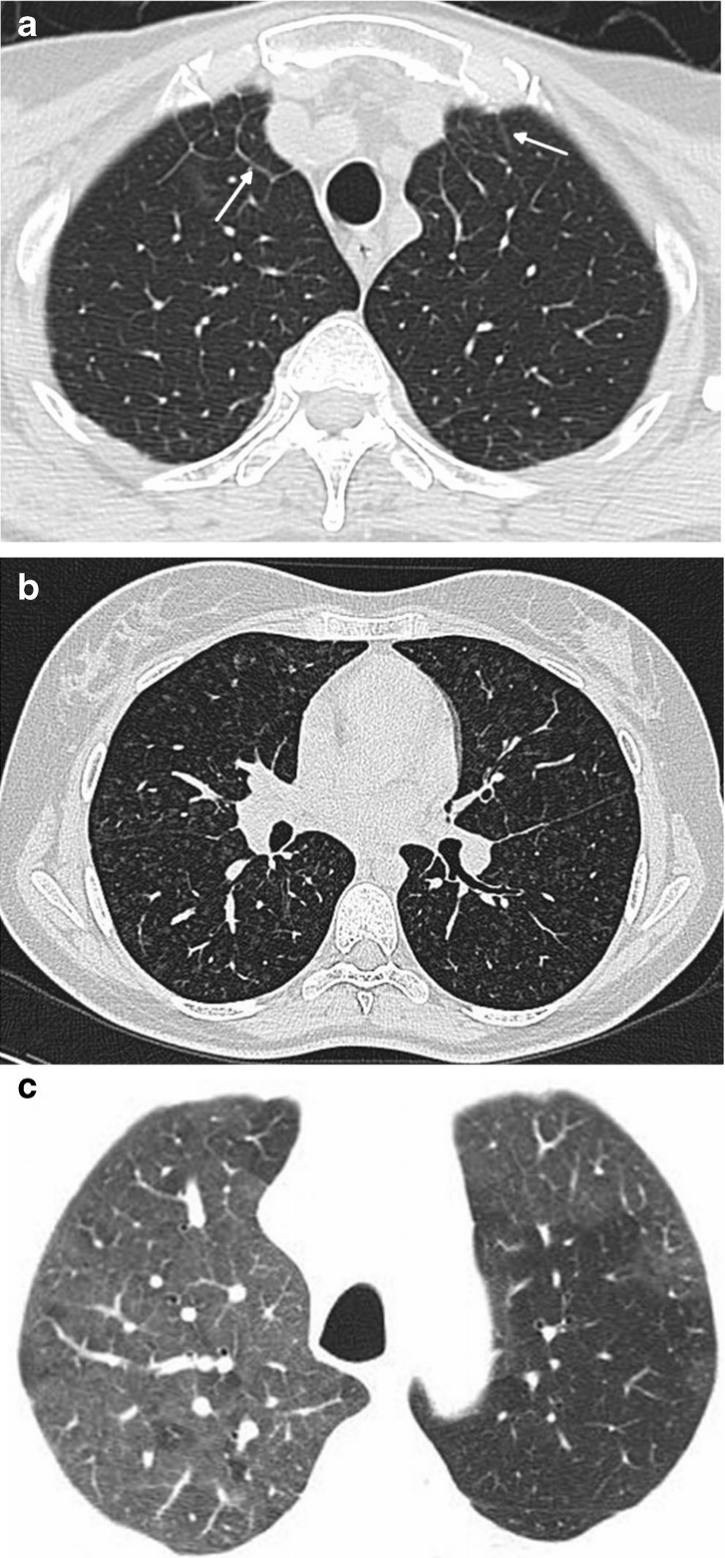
Several patterns of lung infiltration in high-resolution CT scans. a A 73-year-old male with bilateral smooth thickening of the interlobular septa at the lung apices (arrows); b a 39-year-old female with small centrilobular nodules at the mid-portion of the lungs; c a 71-year-old female with patchy areas of ground-glass attenuation at the lung apices
